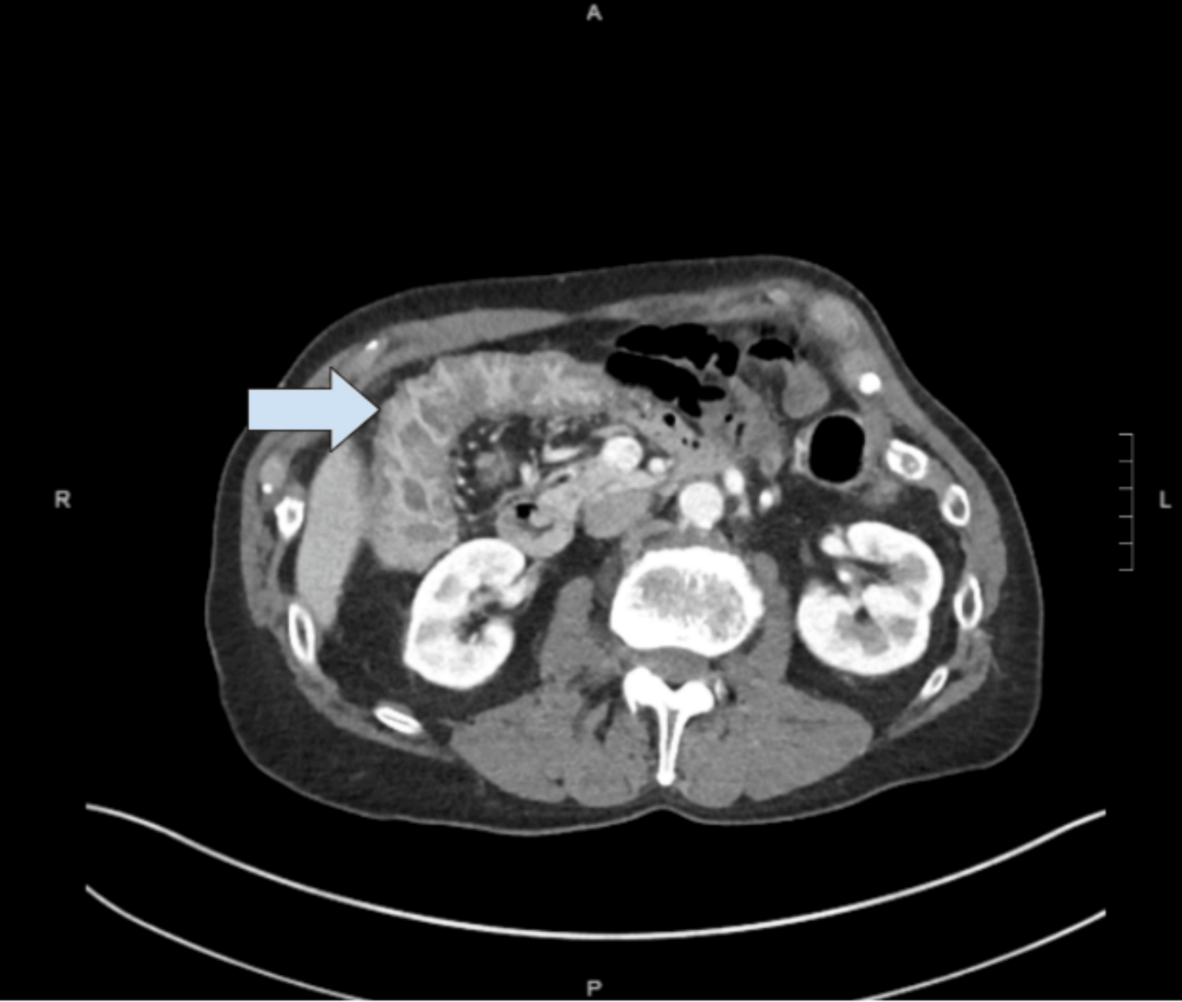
Coronal view of CT scan showing an inflamed transverse colon (blue arrow) as part of the patient's pancolitis
